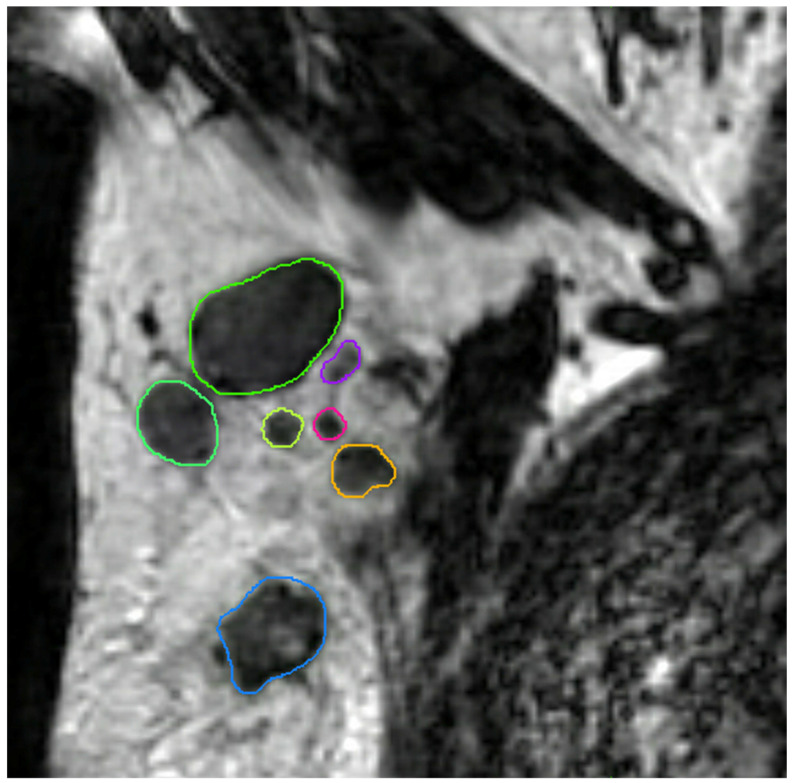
Coronal T2-weighted dedicated axillary MR image of a 55-year old woman with invasive breast cancer, who was treated with mastectomy and axillary lymph node dissection (pT1N2). The MR image demonstrates an example of delineations of lymph nodes in the right axilla on the MIM software.
